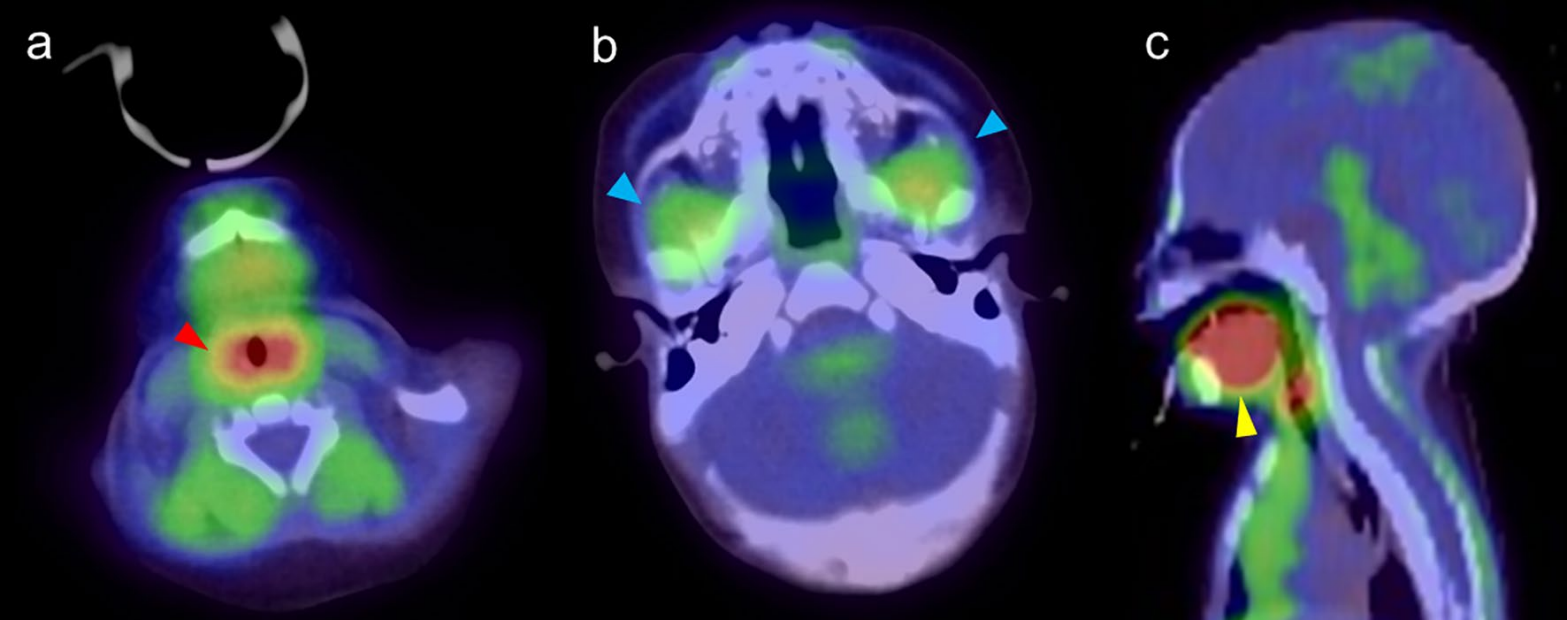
FDG PET/CT image of neonates with15 days after birth. a increased FDG uptake in the muscles associated with vocalization cause by crying (red arrowhead). b increased FDG uptake in masseter used for suck on pacifiers (blue arrowhead). c increased FDG uptake in tongue muscles used for suck on pacifier (yellow arrowhead)
